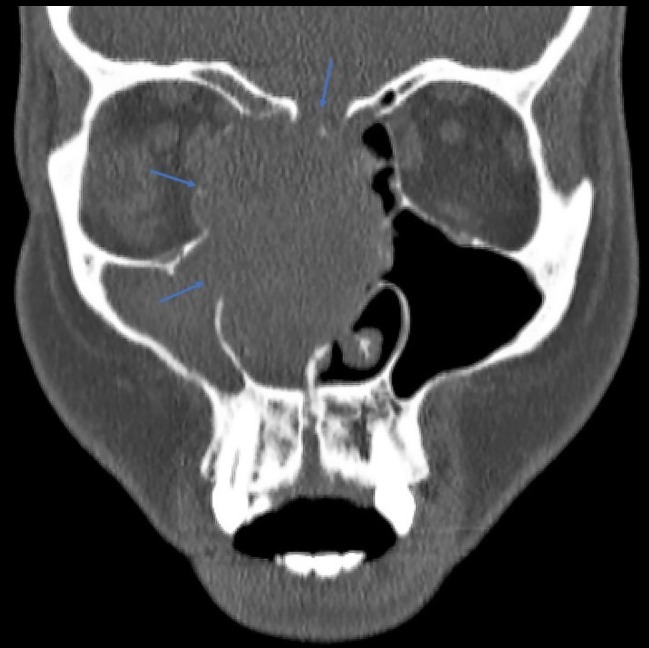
Coronal computed tomography (CT) image demonstrates a 2.6 x 4 x 4.3 cm mass
